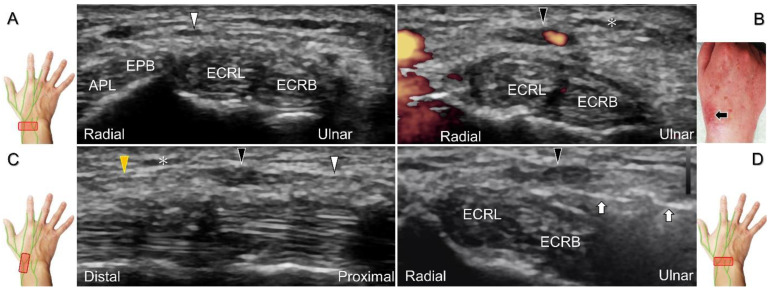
Sonographic imaging of the superficial radial nerve entrapment due to a post-surgical scar. Short-axis imaging at the normal (A) and the swollen (B) segment proximal to the entrapment. The normal, swollen, and entrapped segments of the nerve are seen in long-axis view (C). Ultrasound-guided hydrodissection of the nerve from the ulnar aspect (D). White arrowheads: normal segment; black arrowheads: swollen segment; orange arrowhead: entrapped segment; white arrows: needle; black arrow: scars on the skin; asterisk: scars in the subcutaneous tissue; APL: abductor pollicis longus tendon; EPB: extensor pollicis brevis tendon; ECRL: extensor carpi radialis longus tendon; ECRB: extensor carpi radialis brevis tendon.
